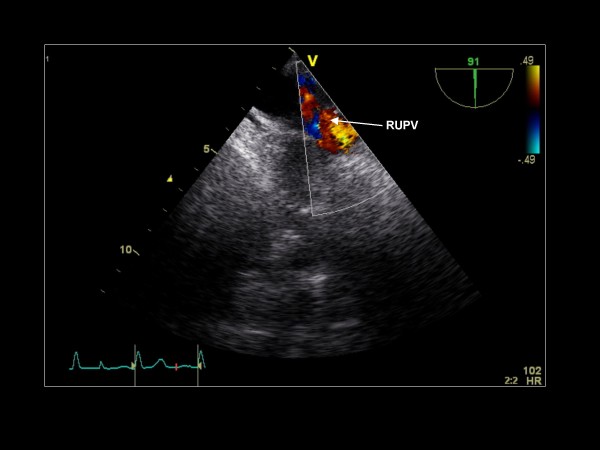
Imaging plane 90°, The right upper pulmonary vein (RUPV) is well visualized with color Doppler.
